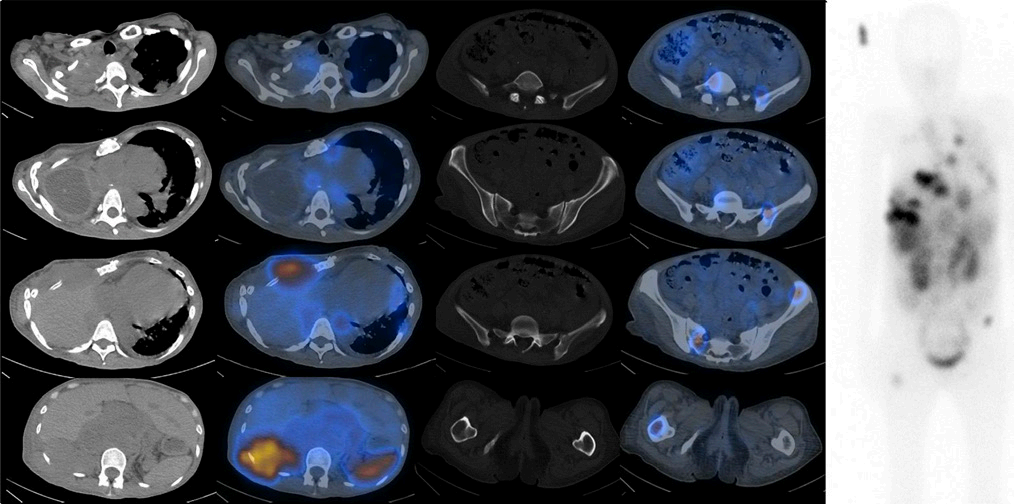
177Lu-DOTATATE SPECT axial images (Left, top to bottom) of right apical pleura, mediastinal adenopathy, right anterior chest wall, right posterior chest wall and retroperitoneal adenopathy, (Middle, top to bottom) fifth lumbar vertebra, left ilium, left iliac crest and right hemisacrum, right femur and Maximal Intensity Projection image (Right) after the second cycle of PRRT.
